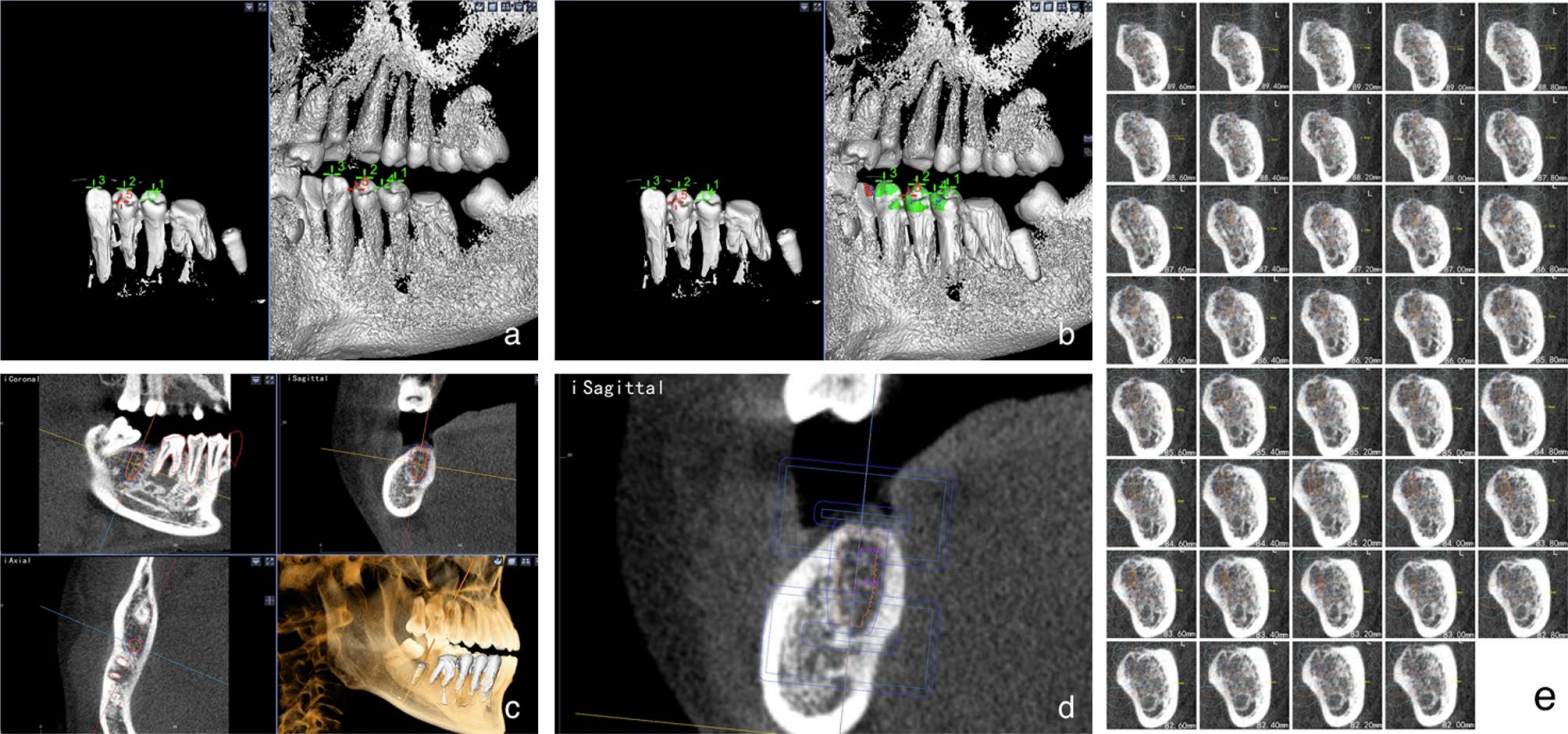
(a) Set registration points based on the cusps. (b) Registered and displayed the postoperative implant position. (c) Virtual implant placement based on actual implant. (d) Divided the implant into three equal parts. (e) Captured each CBCT layer image on the coronal plane.
